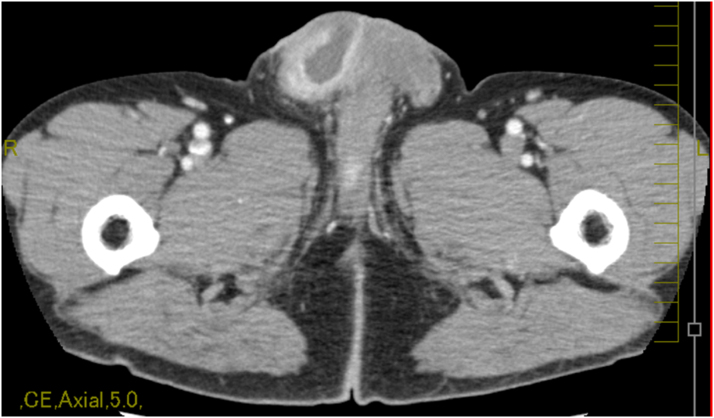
Computed tomography scan showed fluid correction with contrast effect in the capsule of right scrotum.
